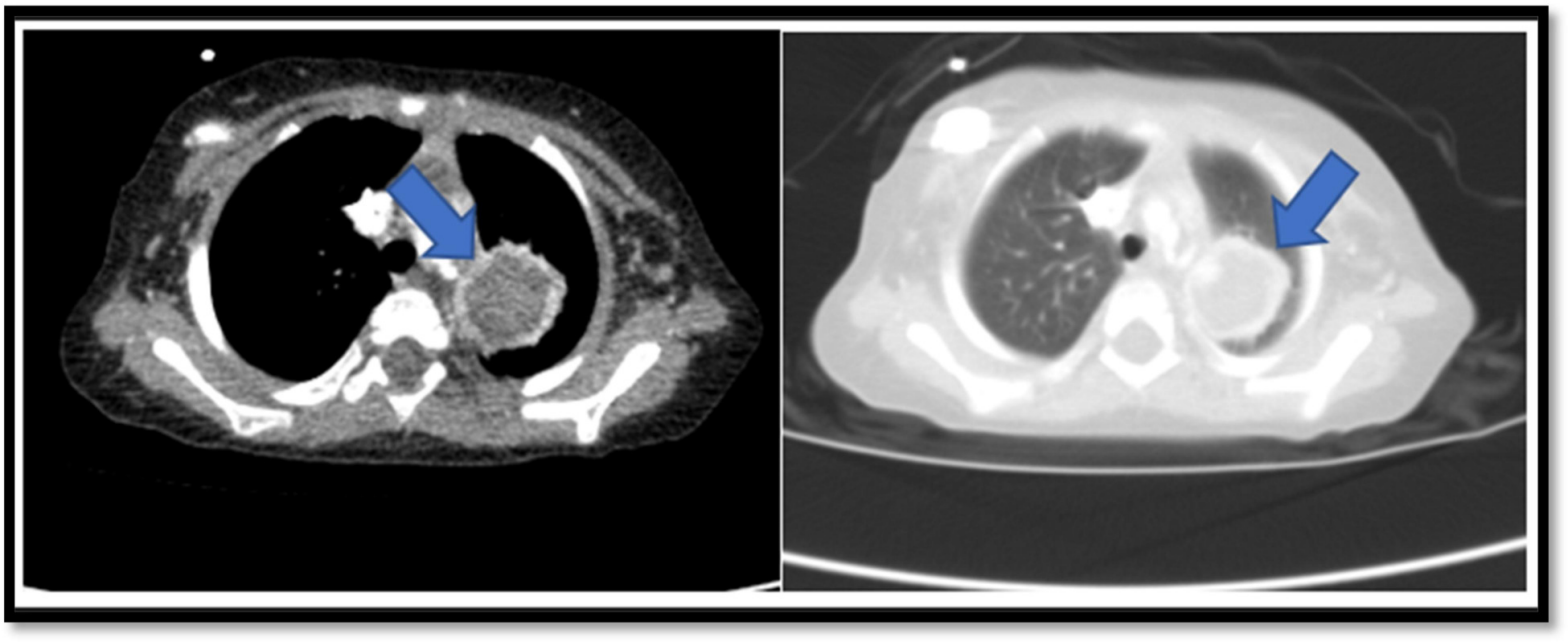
Contrast-enhanced thoracic CT showing a saccular pseudoaneurysm arising from the proximal left subclavian artery. The lesion demonstrates peripheral thrombus, extension into adjacent lung parenchyma, and associated compressive atelectasis—findings consistent with a mycotic pseudoaneurysm due to angioinvasive aspergillosis.
